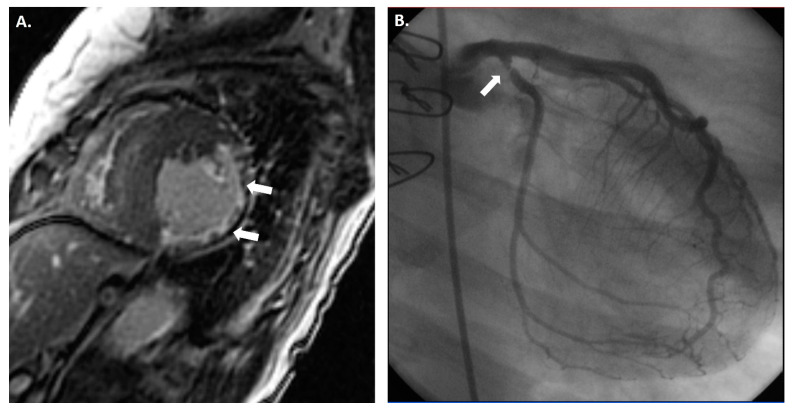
(A) Cardiac magnetic resonance imaging (CMR) showing clear thinning (white arrows) of the anterolateral, inferolateral, and inferior mid-cavity, with subendocardial delayed enhancement in these regions suggesting a vascular etiology. The hypertrophy of the septum is profound but is presumably compensatory due to the extensive loss of lateral wall myocardium. While this CMR finding could be multifactorial, invasive coronary angiography showed a severe obstruction (white arrow) in the proximal circumflex artery (B).
